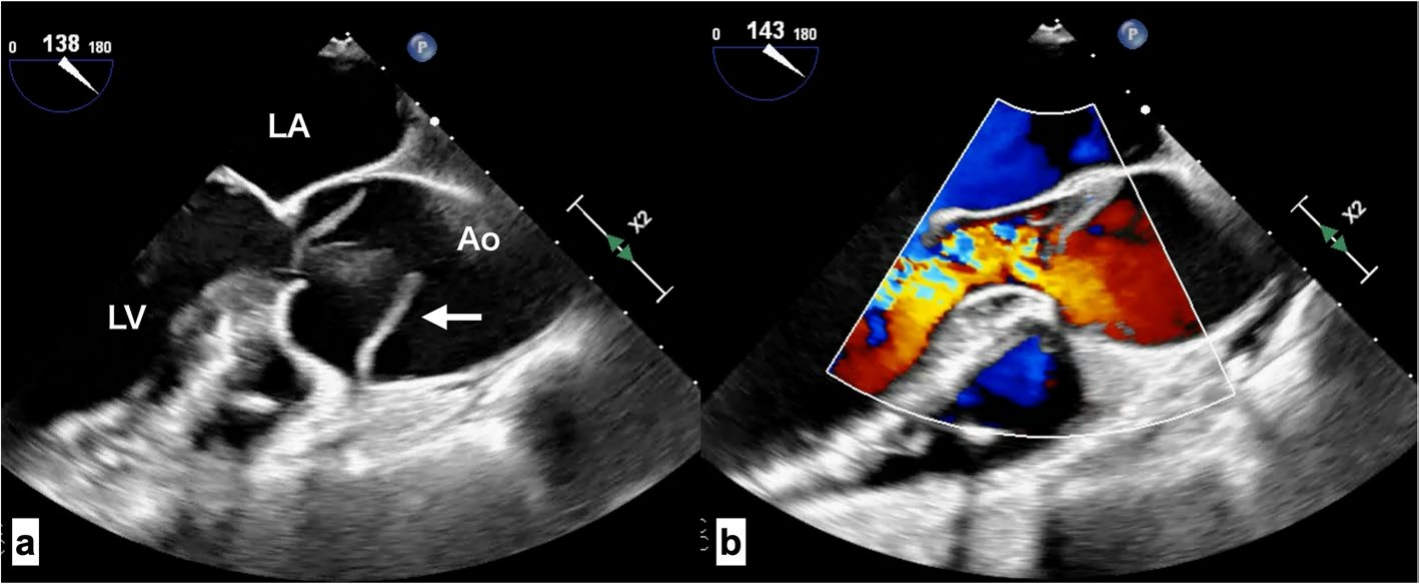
The transesophageal echocardiogram during the surgery shows the intimal flap (a) (white arrow), which is inverted into left ventricle which causes severe AR (b)
